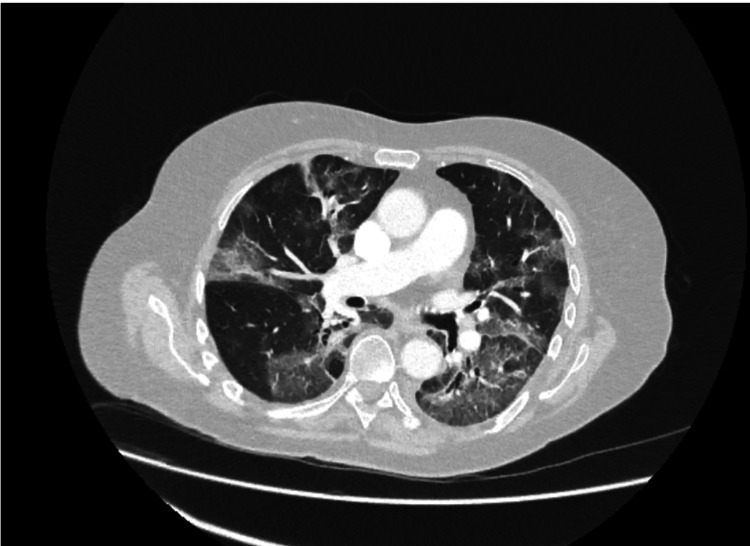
CT of the thorax on day 72 suggestive of Pneumocystis jirovecii infection.
